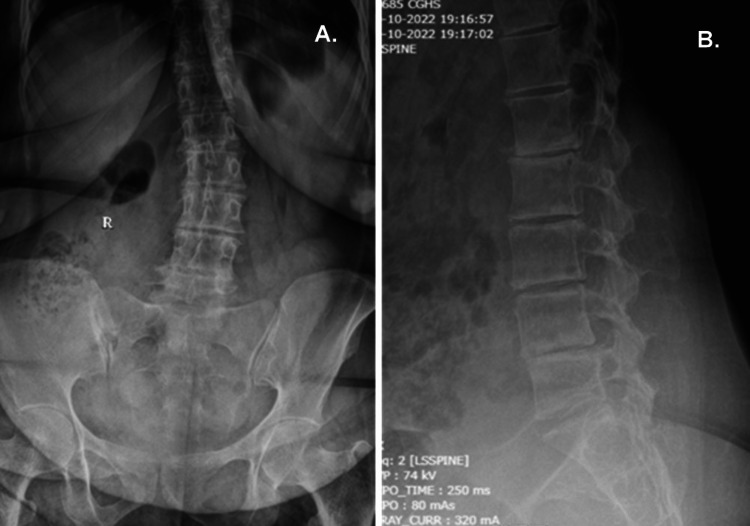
Spine radiographs lumbosacral anteroposterior (AP) view (A) and lateral view (B) showing degenerative changes in the spine
